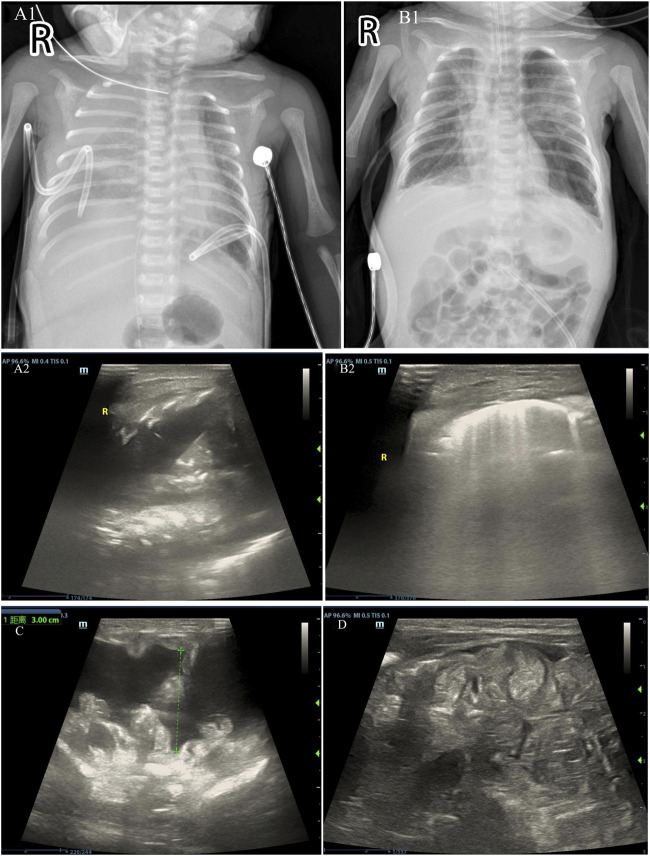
Comparison of Chest Imaging and Abdominal Ultrasound Findings Before and After Treatment. (A1, A2) Pre-treatment chest X-ray/ultrasound showing massive bilateral pleural effusion. (B1, B2) Post-treatment chest X-ray/ultrasound indicating near-complete resolution of pleural effusion. (C) Pre-treatment abdominal ultrasound showing massive ascites. (D) Post-treatment abdominal ultrasound demonstrating marked reduction of ascites. These findings indicate that chylothorax and chylous ascites were significantly improved following comprehensive therapy.
